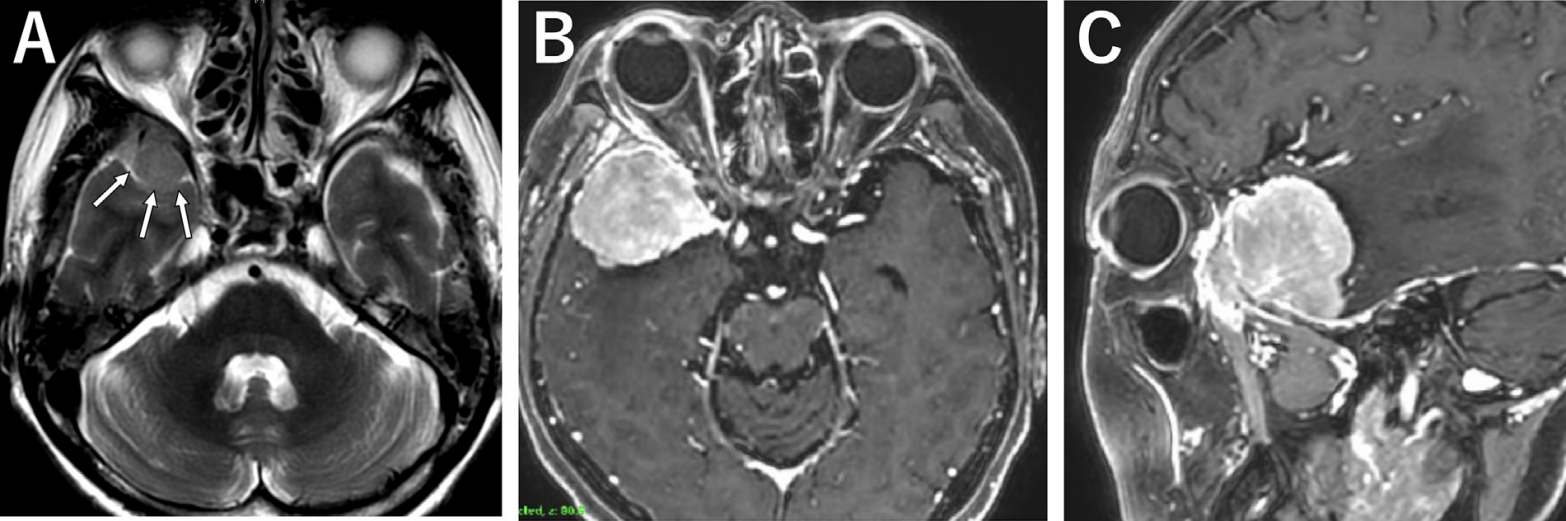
Axial plain T2- weighted MR image 6 years before surgery showing a meningioma at the right-sided sphenoidal wing measuring 15 × 13 × 11 mm (arrows). (A. Contrast-enhancement T1-weighted MR images of axial (B) and sagittal (C) view at the time of surgery showing the tumor measuring 38 × 38 × 41 mm.
